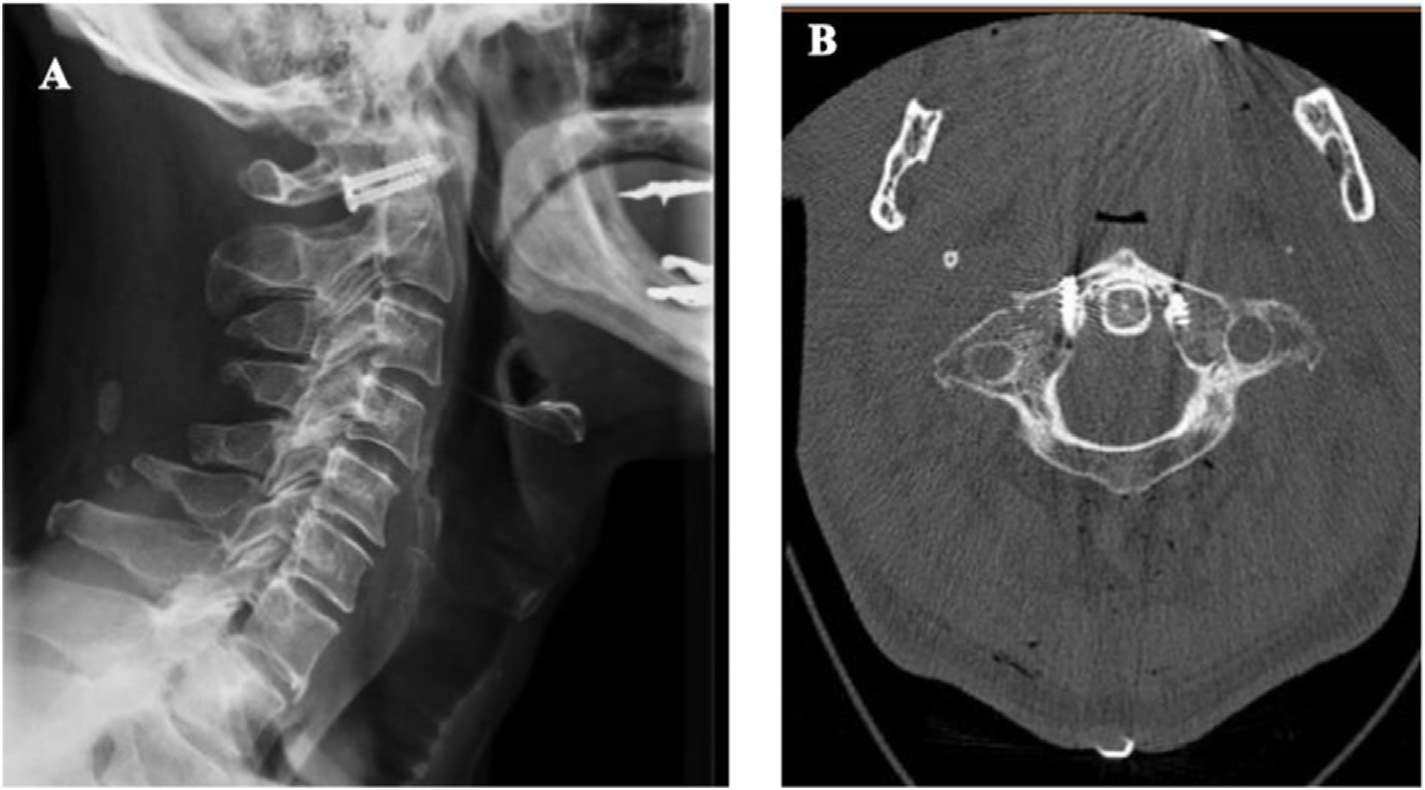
(A–B) RX and CT one day post-op respectively.
